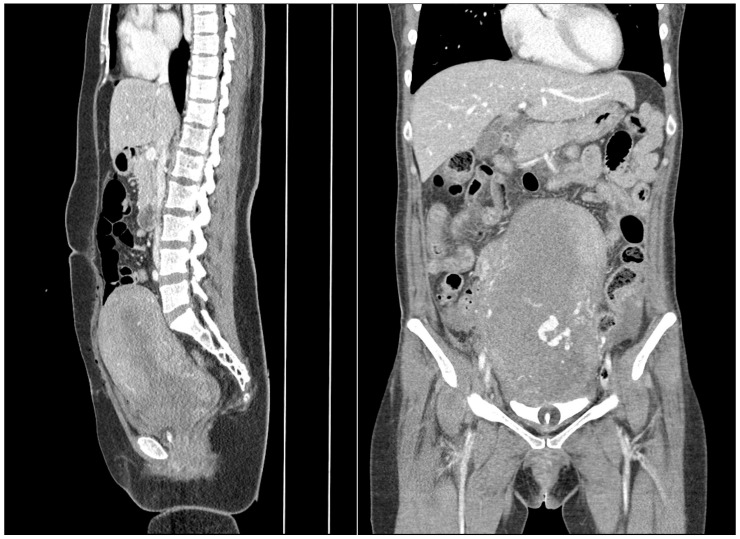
Sagittal (left) and coronal (right) contrast-enhanced computed tomography (CE-CT) images of two patients with postpartum hemorrhage (PPH). (Left) A case without contrast extravasation on CE-CT. The patient was successfully managed with medical treatment including uterotonics and hemostatic agents without the need for surgical intervention. (Right) A case showing definite contrast extravasation in the uterine cavity. Despite initial conservative treatment including uterotonics, hemostatic agents, and uterine artery embolization (UAE), the patient failed to respond and ultimately required surgical management with hysterectomy.
